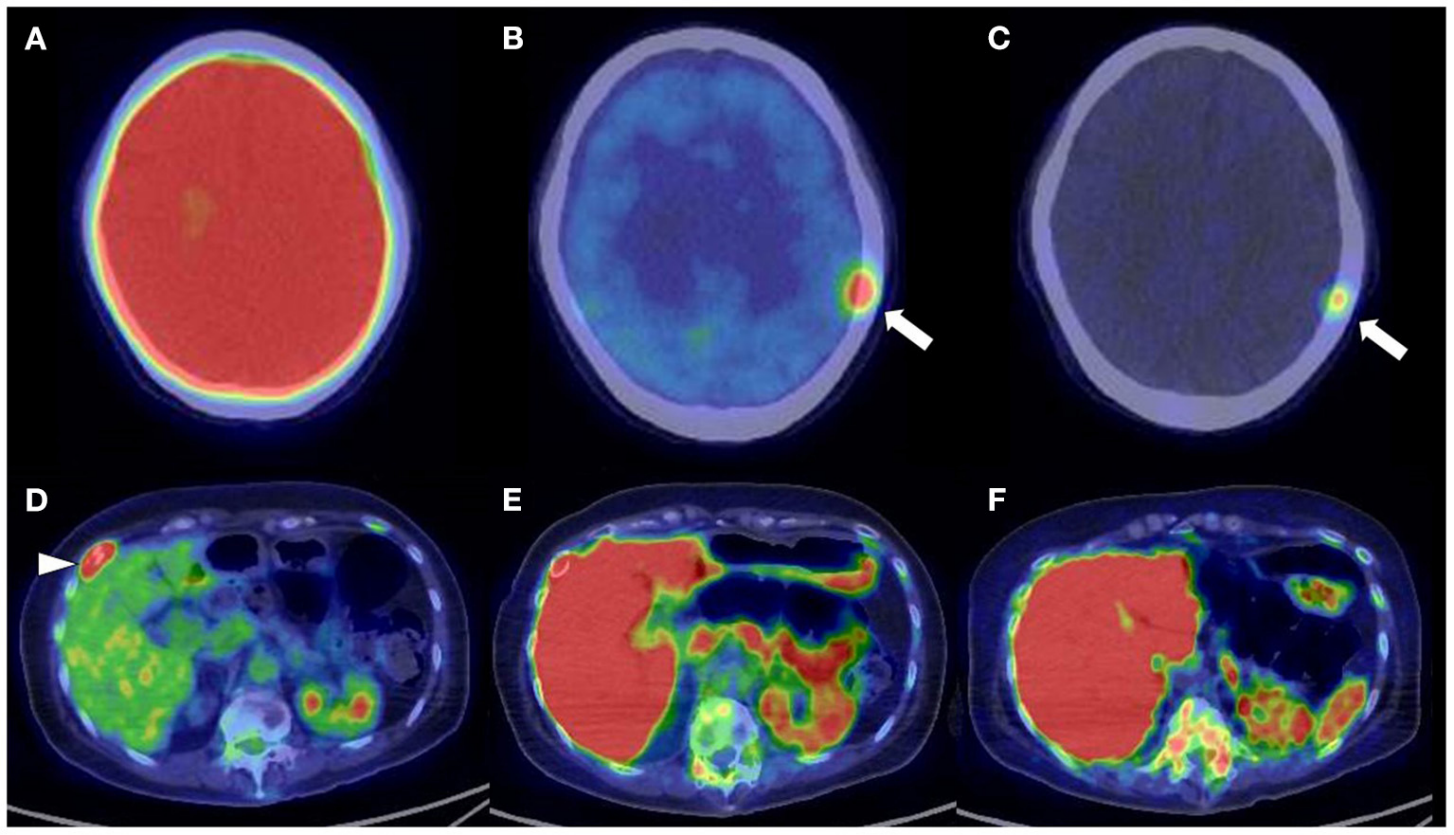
Upper low (cranial bone lesion, *arrow*): (A) 2-[^18^F]-fluoro-2-deoxy-glucose positron emission tomography/computed tomography (^18^F-FDG PET/CT). (B)
